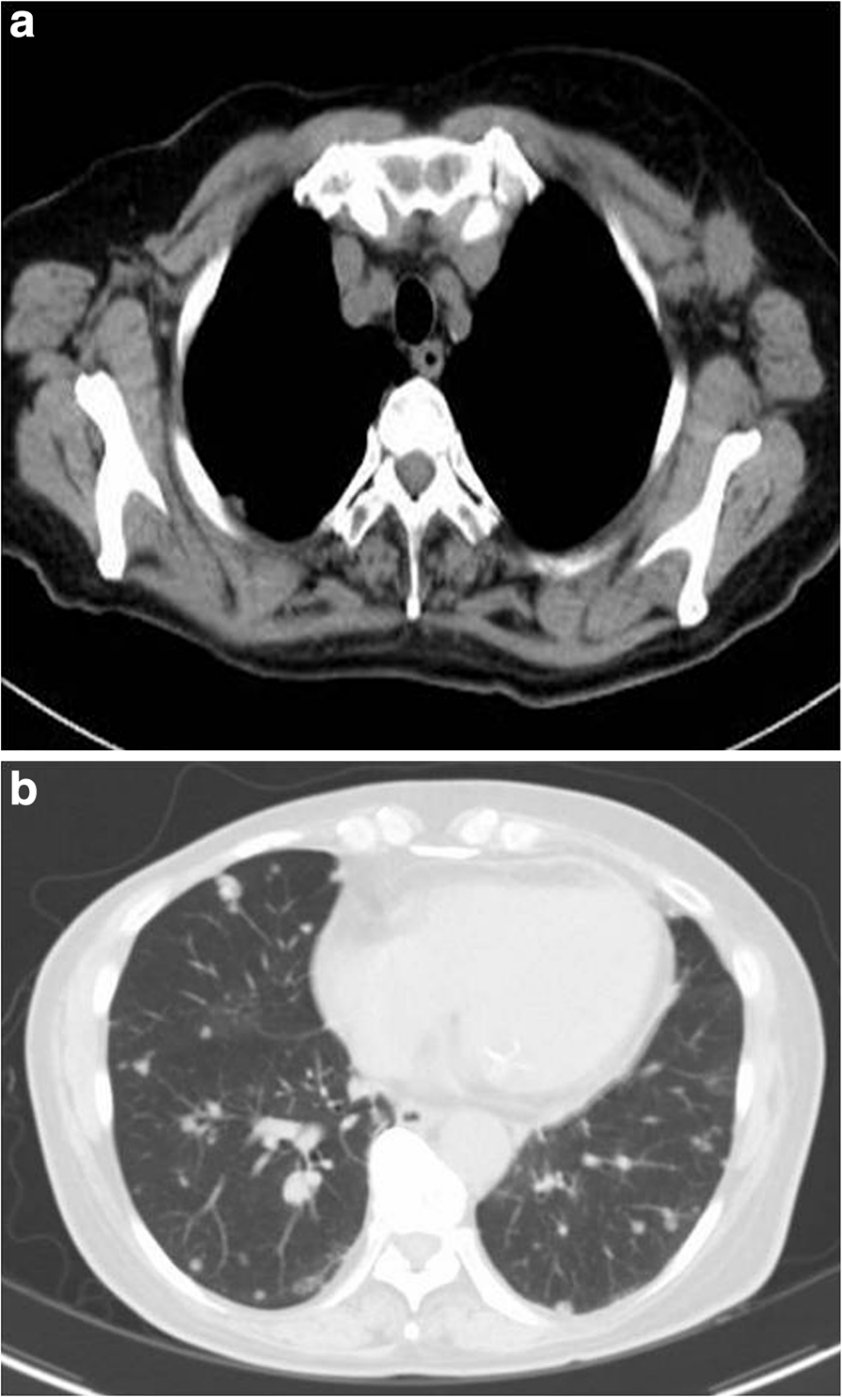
a and b (CT from December 2011). CT showed a left axillary lymph node that had enlarged to 2.5 cm and lung metastases that had also enlarged.
